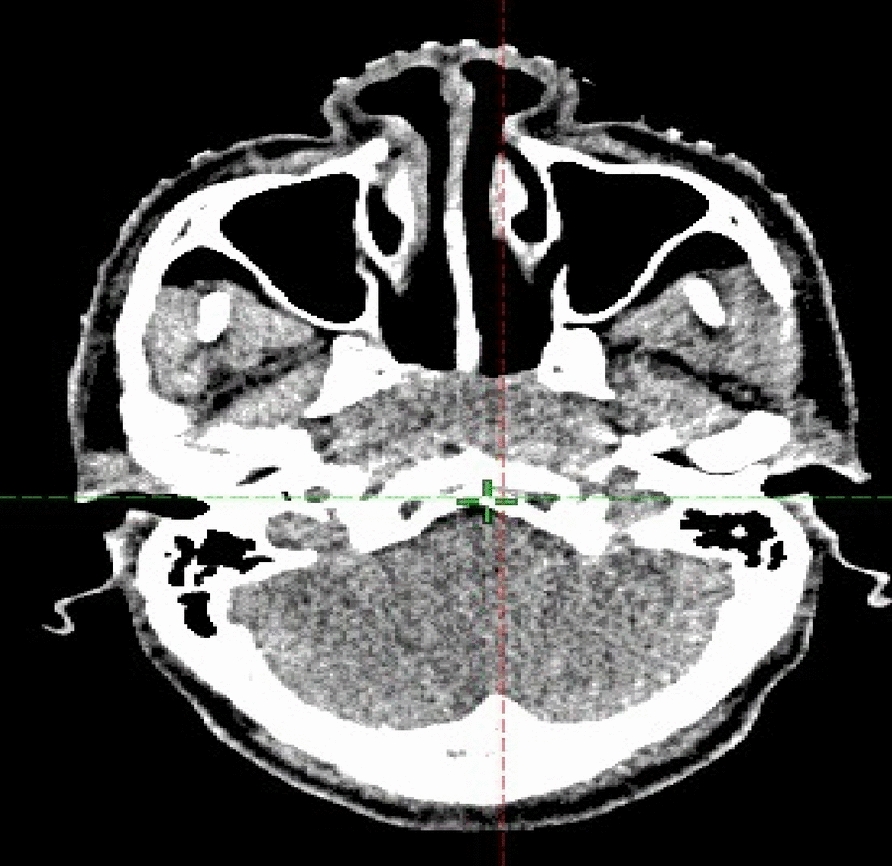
Computed tomography scan after three cycles of chemotherapy
